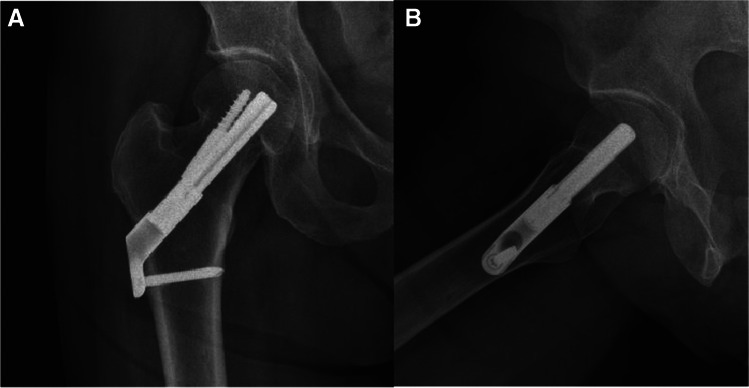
A, B AP and lateral radiographs from last follow-up, 24 months after the surgical treatment. Walks unaided, no complaints of chronic hip pain
